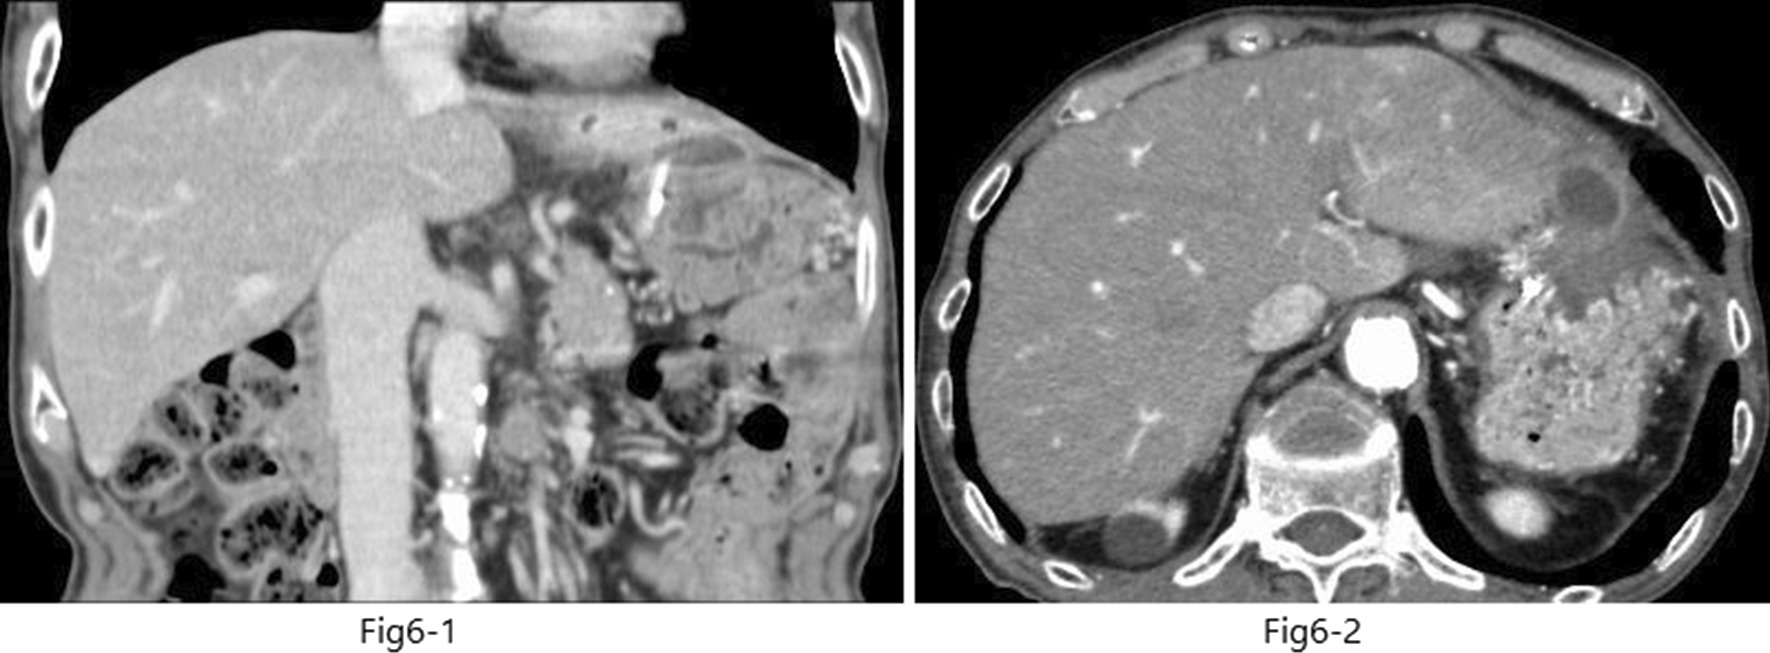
(1) Computed tomography scan (coronal view) showing that the abscess is smaller in size. (2) Computed tomography scan (axial view of the same image as in 1)
